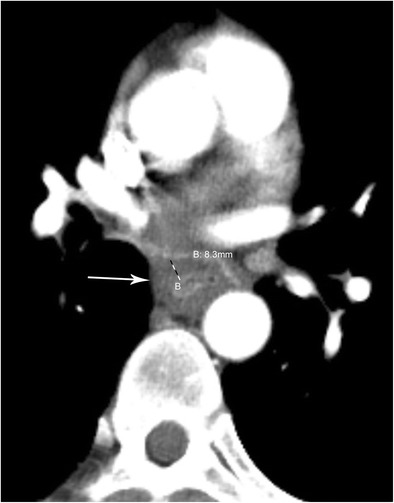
Oesophagitis. CT angiogram of the chest in soft tissue window shows circumferential oesophageal wall thickening in a patient who presented with chest pain. Please note the contrast enhancement of the mucosa which creates a hyperdense inner layer contrasting with the rest of the wall; this is an imaging feature that can be observed in gastrointestinal inflammatory diseases
